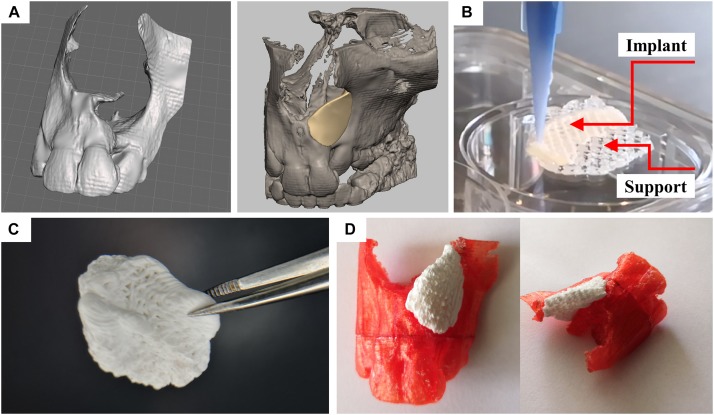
Design and fabrication process of a patient-specific implant consisting of a calcium phosphate cement. (A) Three-dimensional reconstruction of a CT scan of a patient and designed model of a perfectly fitting implant. (B) Fabrication process by multichannel 3D plotting of a sacrificial ink and the CPC. (C) Photograph of the implant, after the sacrificial ink was washed away. (D) The hardened implant fits perfectly into the defect of the patient. Red: Plastic model of the maxilla with incisors, white: 3D plotted CPC implant.
